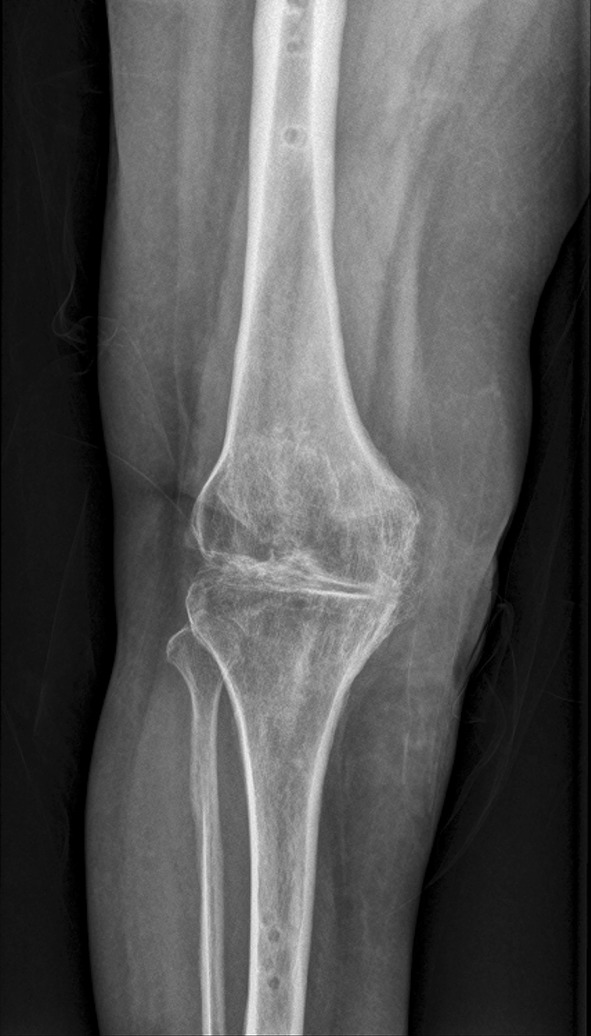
Antero-posterior plain radiograph at seven months with consolidation at the arthrodesis site
